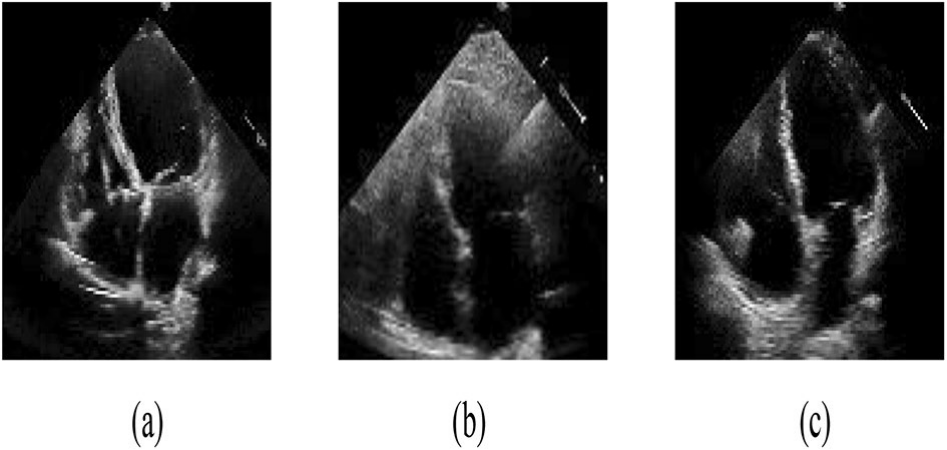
Echocardiograms showing (a) ES phase (b) ED phase (c) Non-ESED frame.
